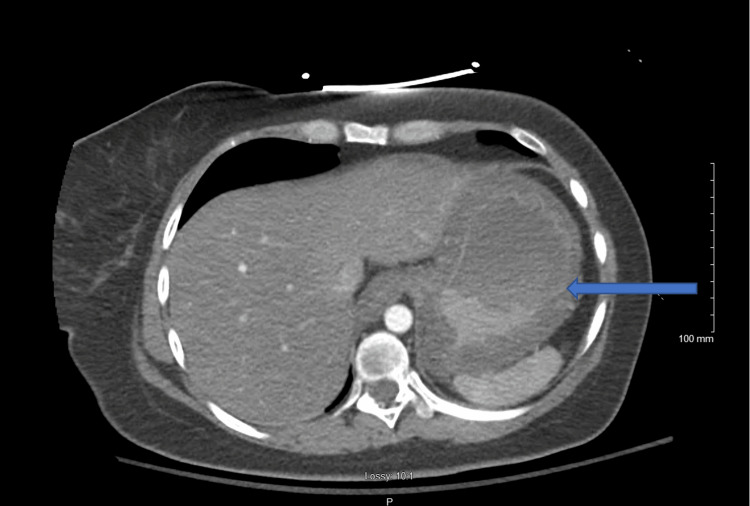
CT abdomen and pelvis with intravenous contrast with arrow signifying marked diffuse thickening of the stomach.
